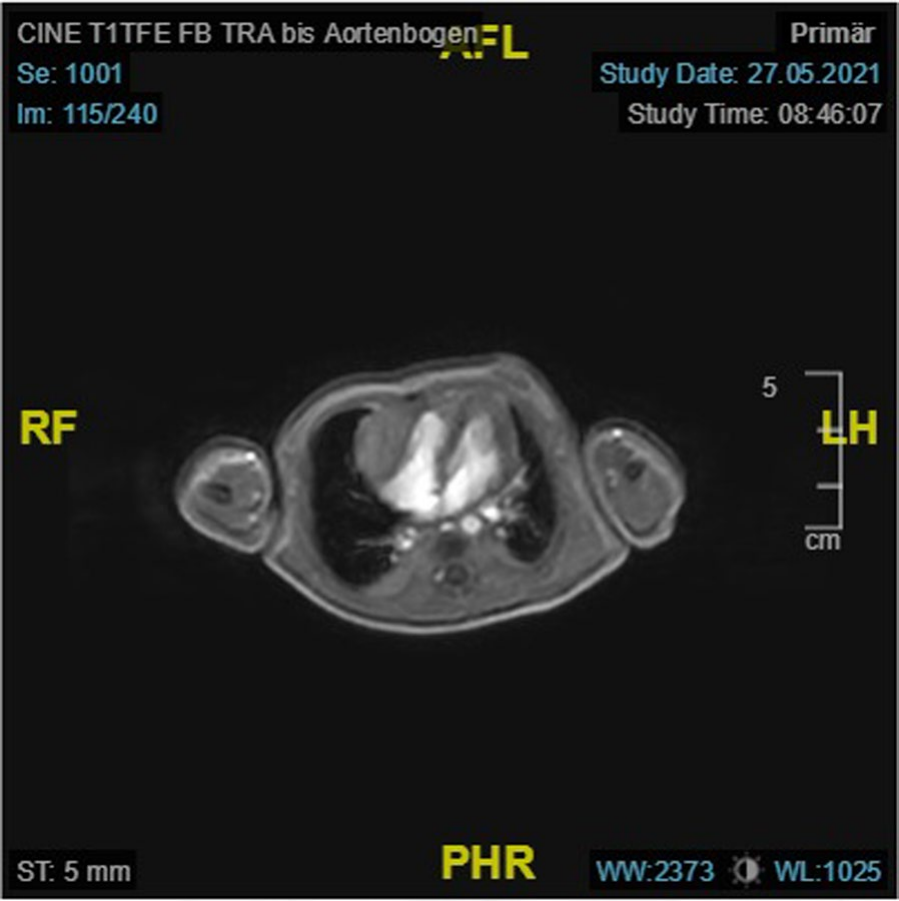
Cardiac magnetic resonance imaging. Legend: Cardiac magnetic resonance imaging showing an isointense mass on the lateral wall of the right ventricle in the T1-weighted sequence
